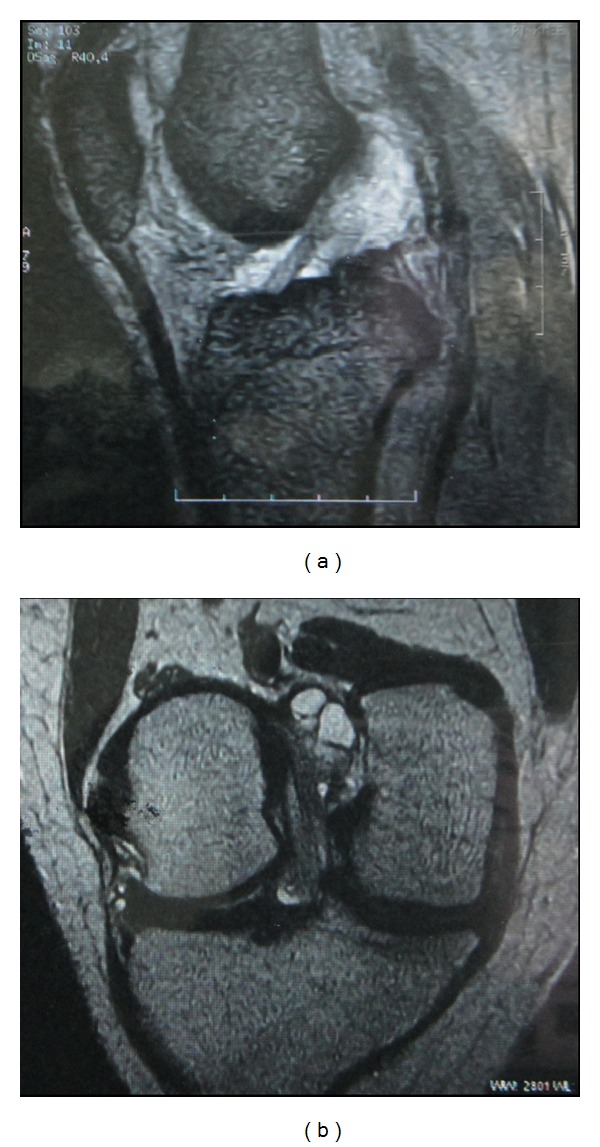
MRI images of ACL ganglion cysts.
